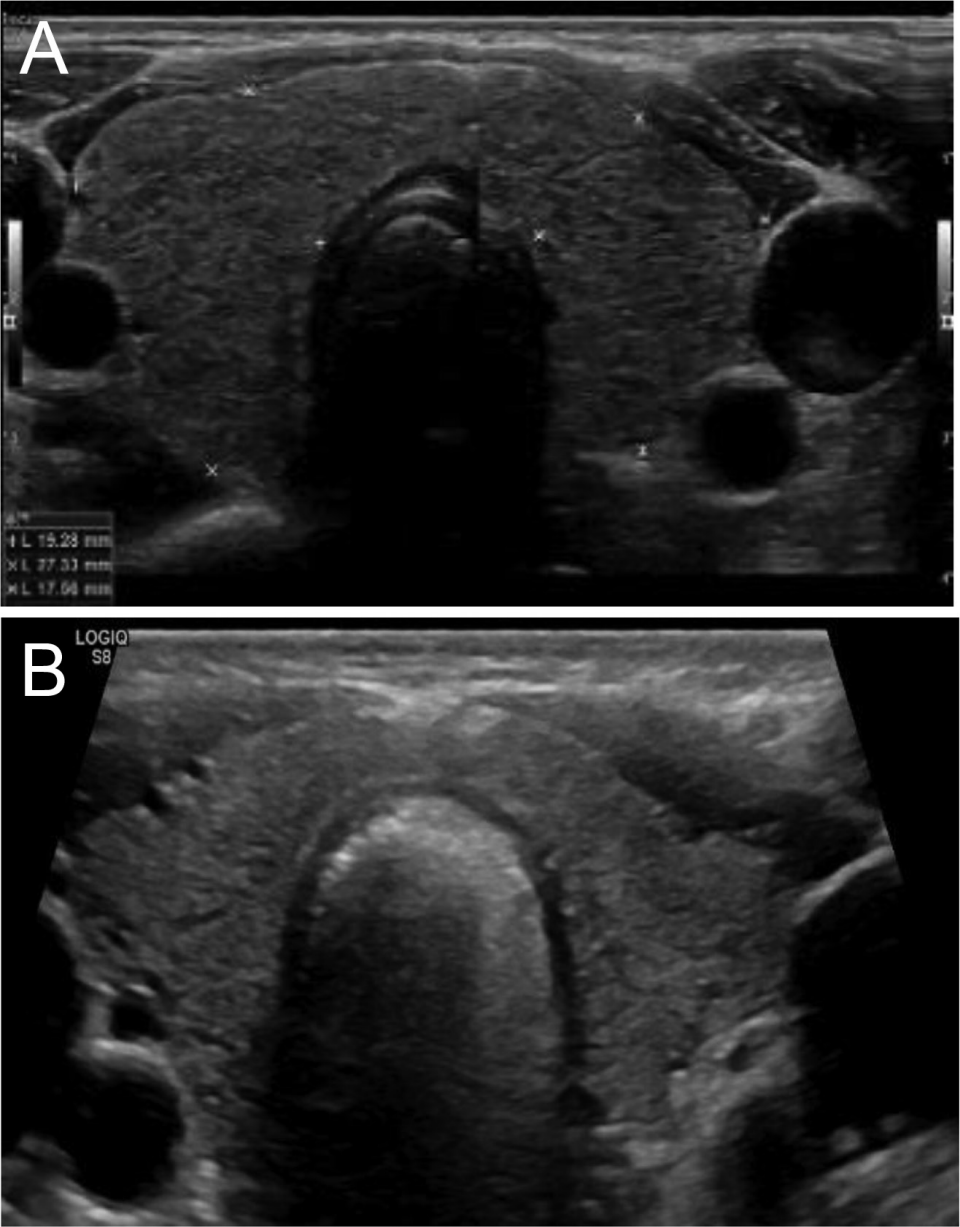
Thyroid ultrasound performed before (A) and 18 months after thyroid embolization (B) depicting a volume reduction of the right lobe of 44%, of the left lobe of 58%, and of the isthmus of 44%.
